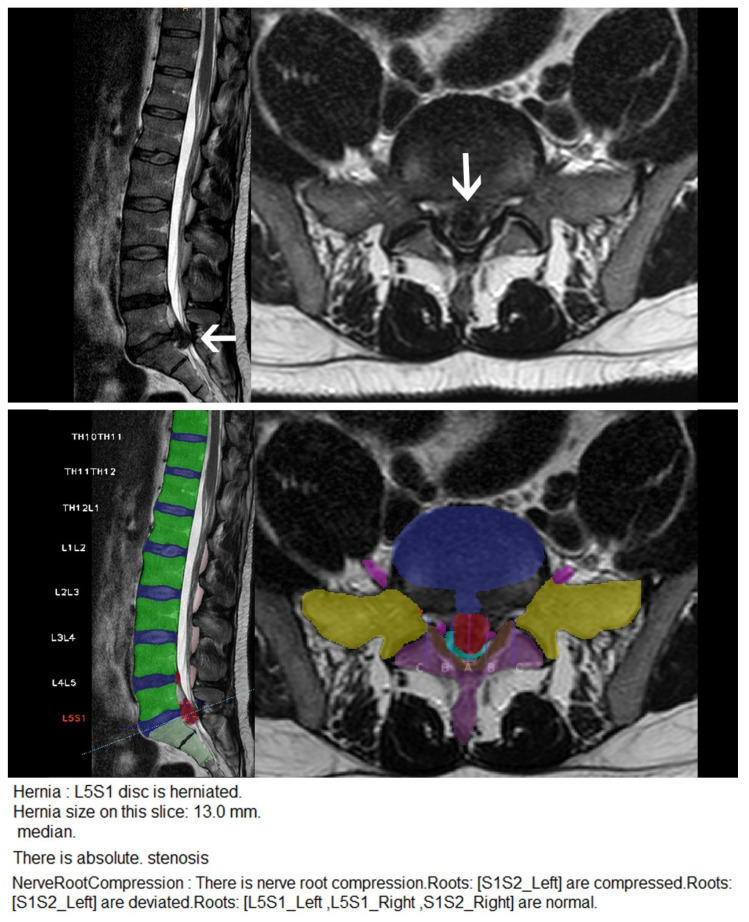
Disc extrusion and spinal canal stenosis correctly detected by the CNN. Upper row: Sagittal and axial T2 weighted MR images of the lumbar spine showing a large disc extrusion (arrows) with severe spinal canal stenosis. Middle row: User interface of the CNN. Each segment of the lumbar spine is correctly labelled by the CNN with the segment showing the most severe pathology being highlighted in red. The vertebral bodies are highlighted in green; the lumbar discs are highlighted in blue. The transverse processes are highlighted in yellow; the laminae and the spinous processes are highlighted in purple; the flava ligaments are highlighted in brown. The dural sac is highlighted in light blue, the nerve roots are highlighted in pink. Disc bulgings and disc herniations are highlighted in red. Lower row: Excerpt of the written report automatically generated by the software.
